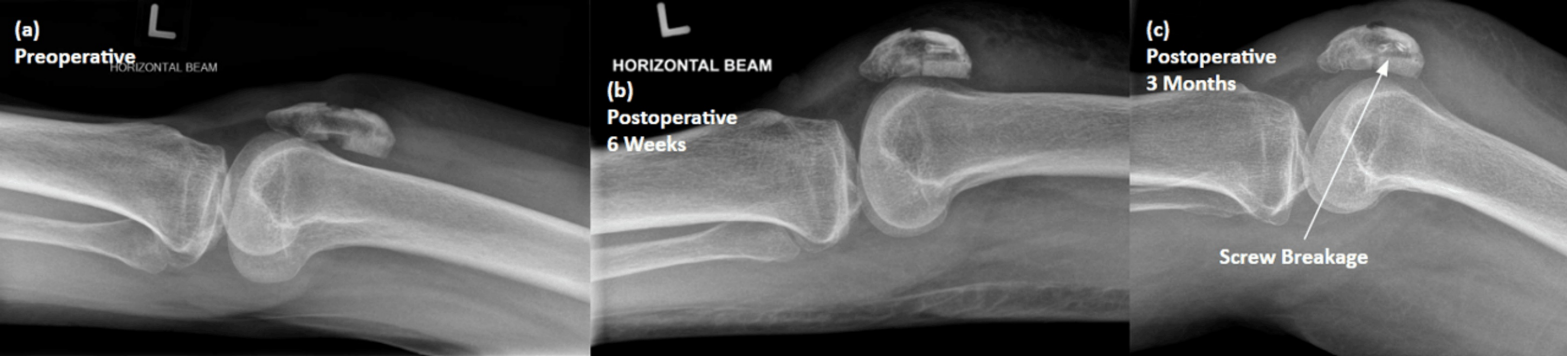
Radiograph of a mid-pole transverse patellar fracture fixed with a resorbable screw-augmented suture technique (A) Preoperative X-ray showing the mid-pole displaced transverse patellar fracture (B) Six weeks postoperative X-ray after hybrid fixation of the patellar fracture (C) Three months postoperative X-ray showing breakage of the screw, new since the previous radiograph, though there is interval healing of the fracture
